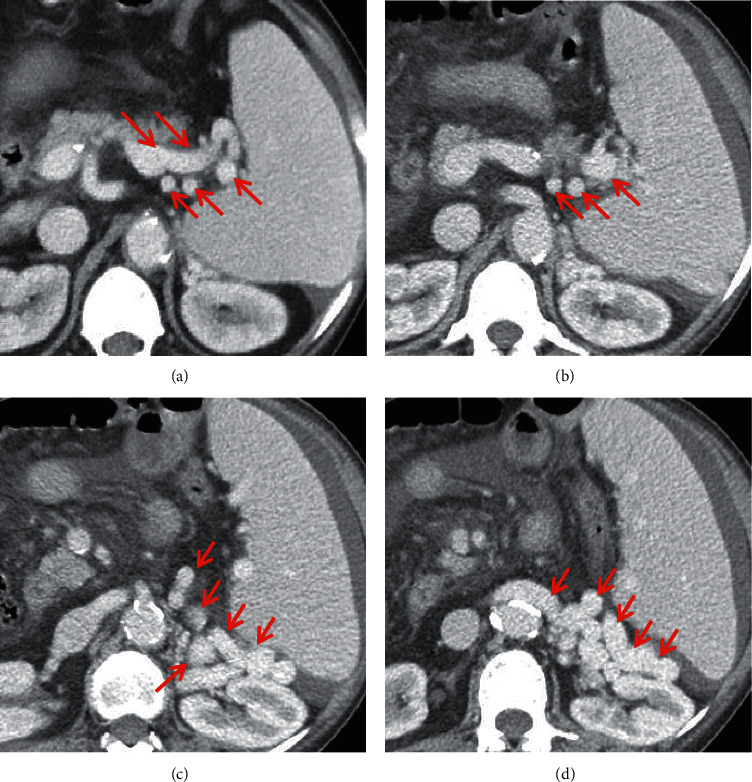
Splenorenal shunt. In a 62-year-old female with liver cirrhosis, the axial contrast-enhanced CT scan on the portal vein phase demonstrated that splenorenal shunt (red arrow) manifested as enhancement of abnormally dilated vessels originating from the splenic vein to the left renal vein.
